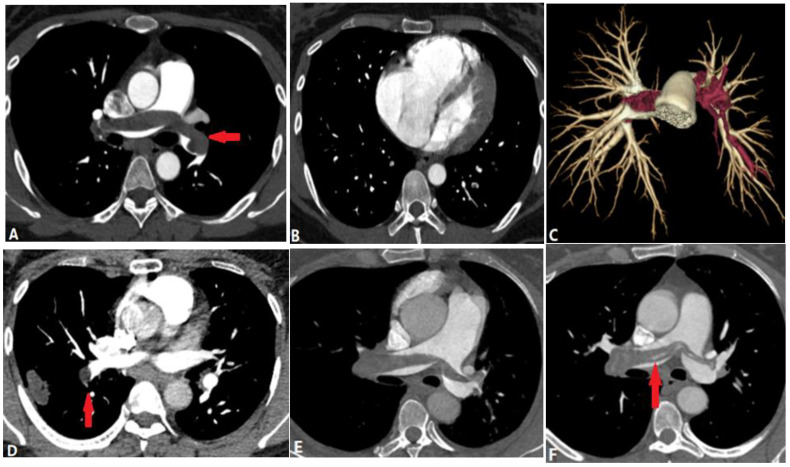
Pulmonary thromboembolism. (A): Axial CT image shows a partial filling defect in the left and right pulmonary arteries, which is non-enhanced and surrounded by contrast (arrow). (B): Axial CT image shows enlargement of the right ventricle and atrium (RV:LV ratio > 1), and 3D volume-rendering image (C) shows the range of the thrombus, which is marked in red. (D): Axial CT image shows the complete filling defects of another patient, with no enhancement of the entire lumen (arrow) and subpleural pulmonary infarction. (E): Axial CT image of another patient who underwent thrombolytic therapy shows partial filling defects in the pulmonary artery. (F): After 4 months’ treatment, there are some band-like enhancements (arrow) in the thrombus, indicating thrombolysis. LV—left ventricle; RV—right ventricle.
